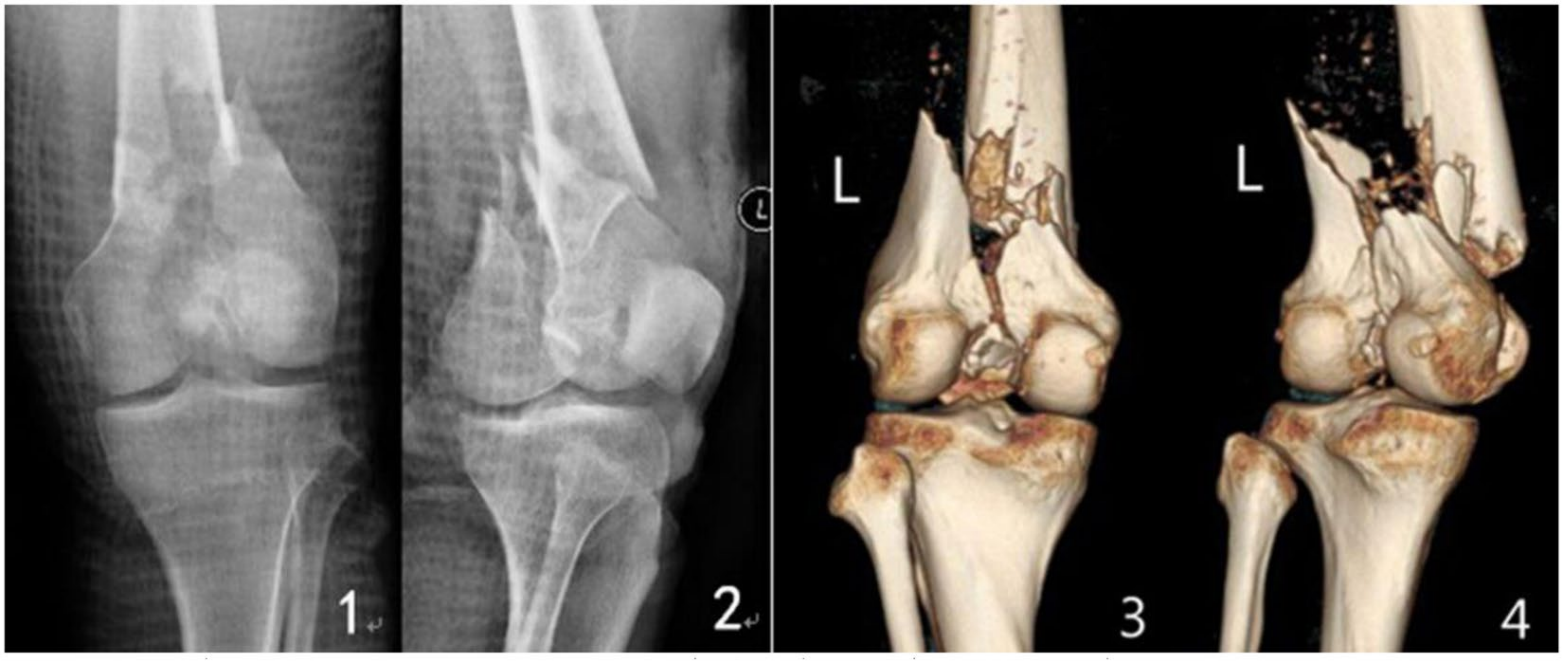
1, 2, 3, 4 the preoperative X-ray and CT three-dimensional reconstruction of femur.
